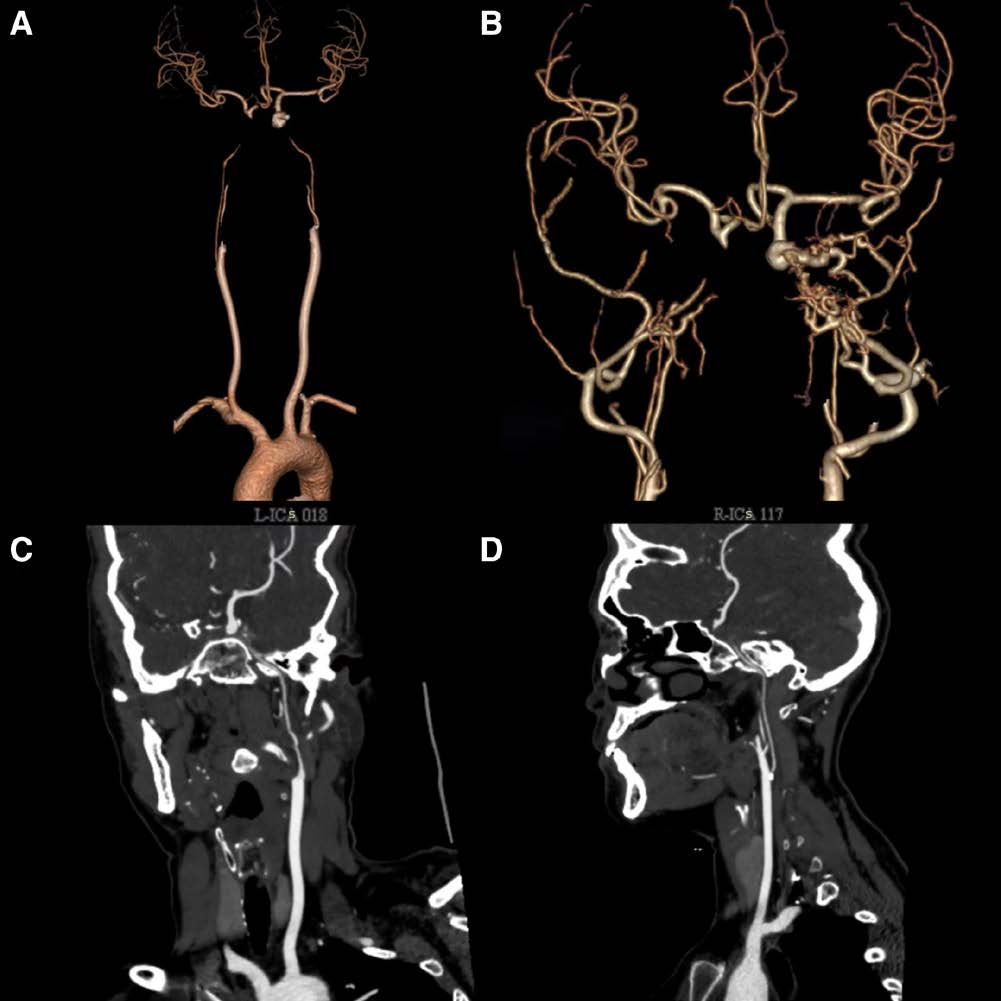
CT angiography showing bilateral internal carotid arteries were small and dysplasia, main trunks disappeared at the cavernous sinus. CT angiography = computed tomography angiography.
